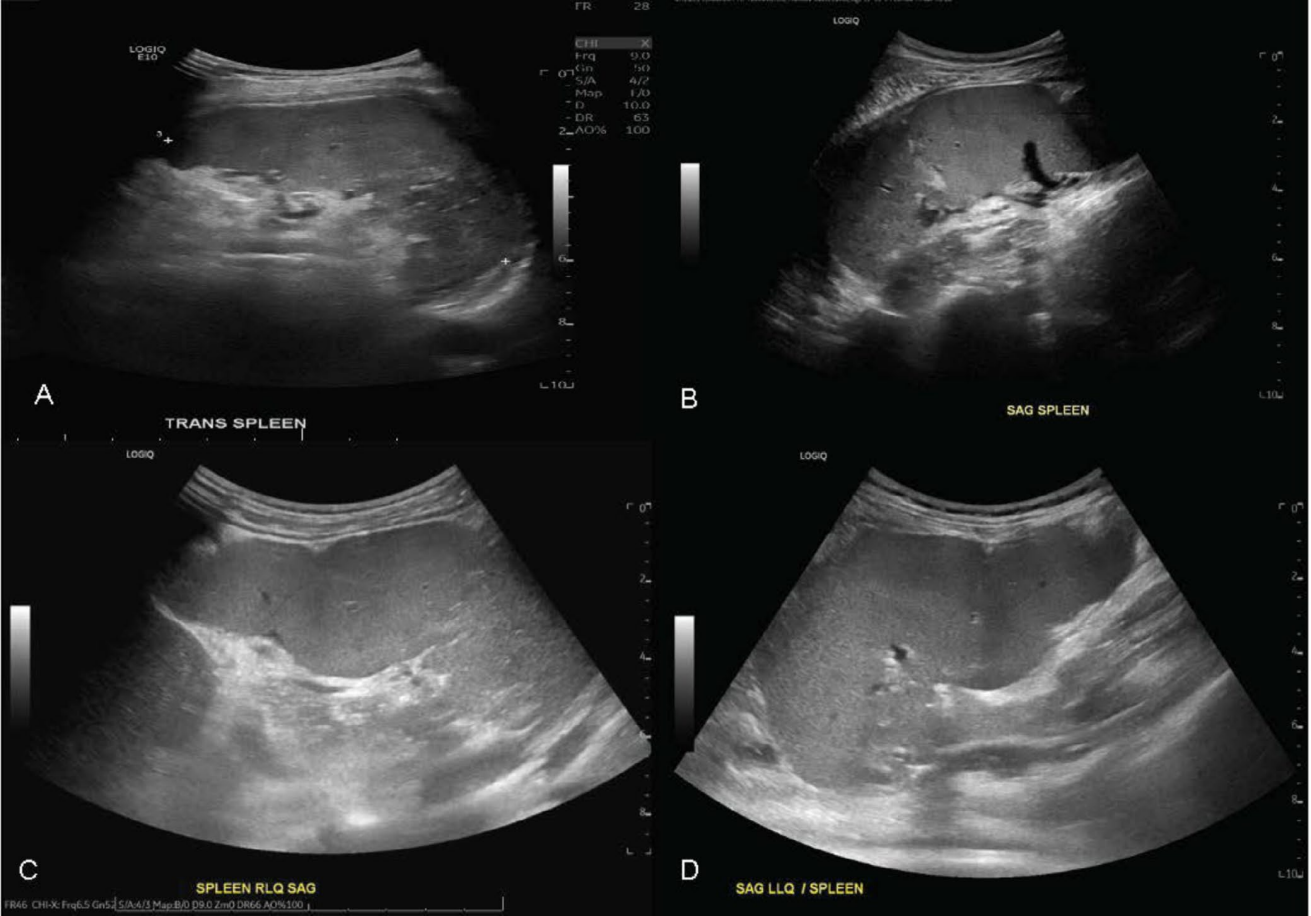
(A) Abdominal ultrasound showing heterogeneous echotexture in the inferior portion of the spleen without internal blood flow measured by Doppler (not shown), consistent with splenic infarct. (B) Abdominal ultrasound which shows normal spleen in correct anatomical positioning. (C) Abdominal ultrasound shows the spleen as “wandering” and located in the RLQ. Splenic blood flow measured by Doppler (not shown) was intact; though there was evidence of decreased flow velocity or vascular congestion. (D) Abdominal ultrasound showing wandering spleen now located in the LLQ. The ultrasound again demonstrated intact blood flow via Doppler (not shown) though vascular congestion.
